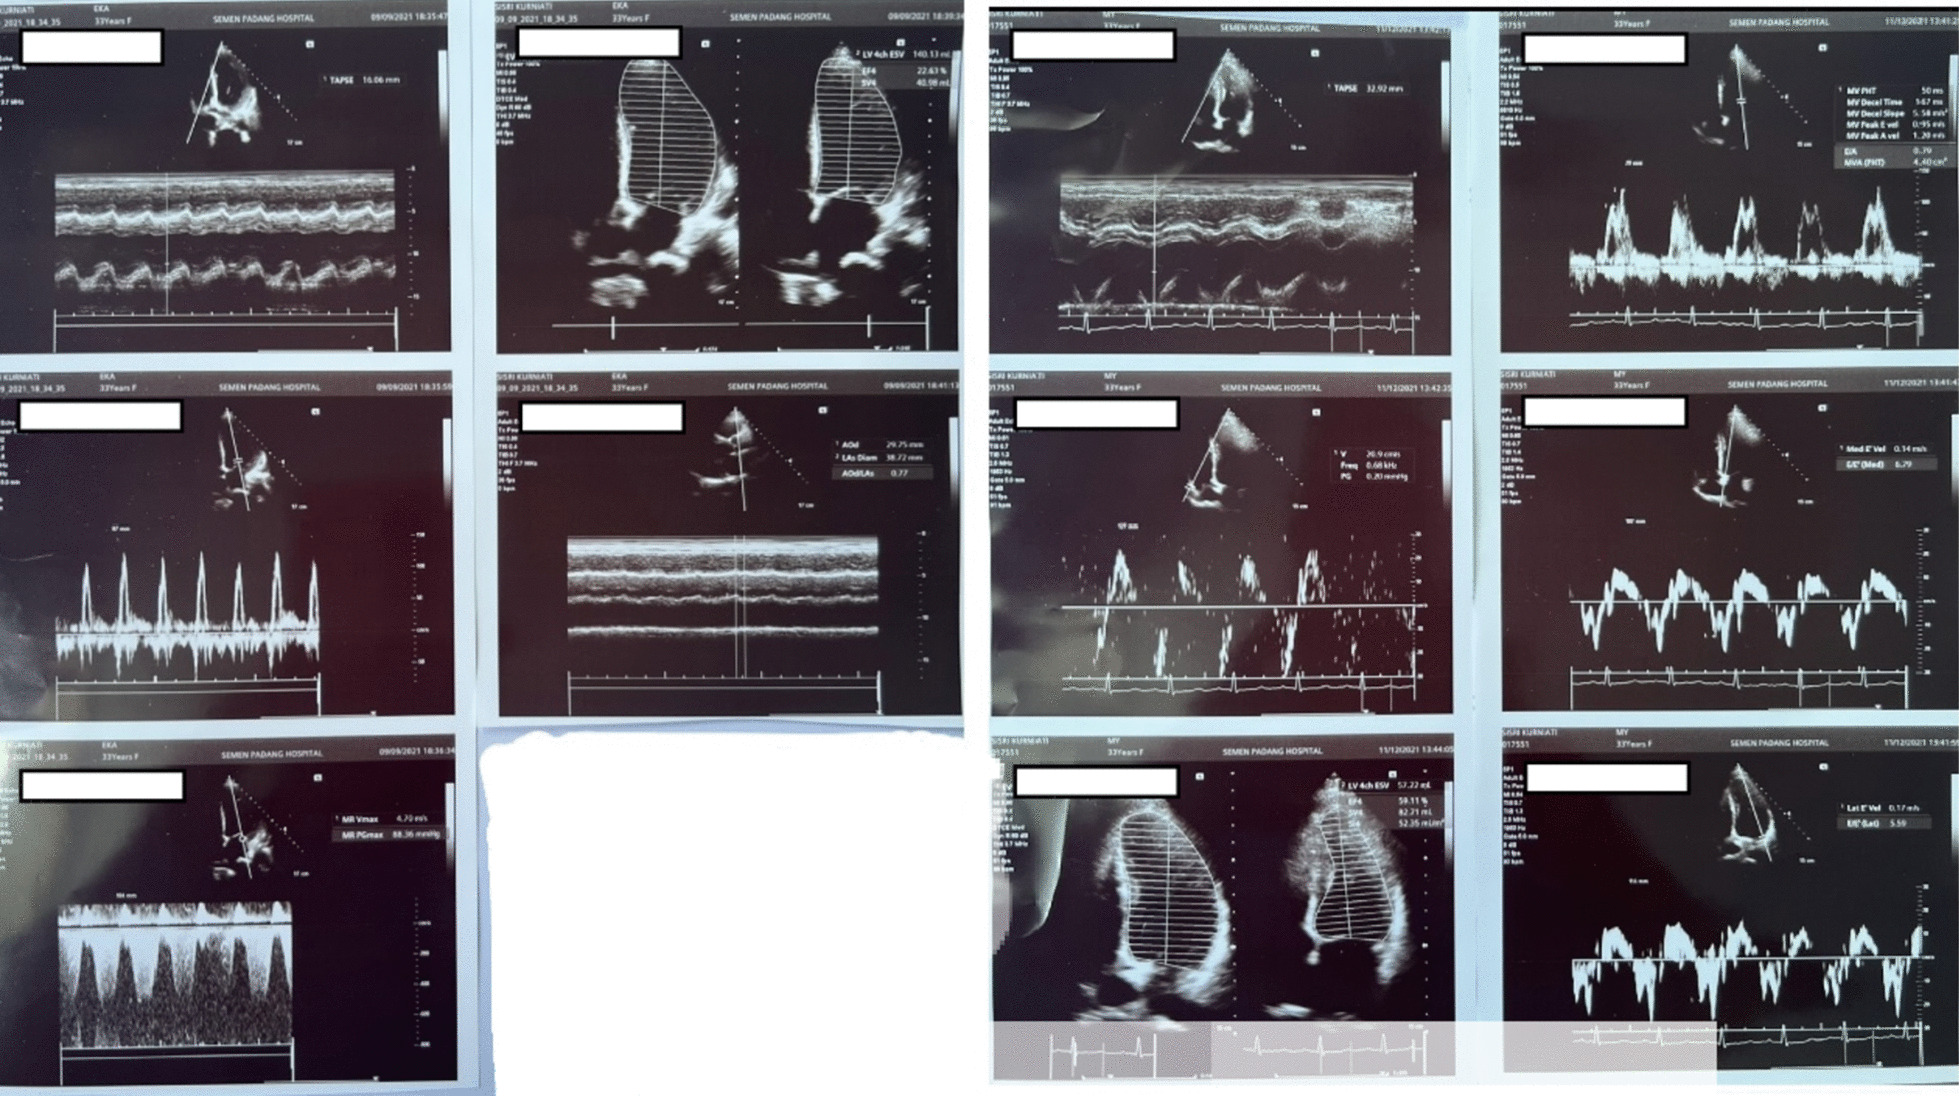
Echocardiography examination (left: post-operative day 0, right: 6 months later)
